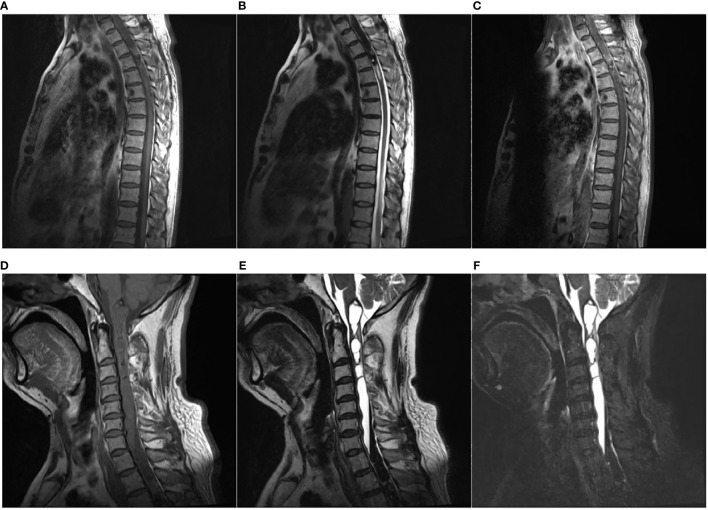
Magnetic resonance imaging (MRI). (A–C) An intramedullary tumor was located at C6–T4 with mixture of iso- and hypointensity on sagittal T1-weighted image (T1WI) (A), non-homogeneous hypointensity on sagittal T2-weighted image (T2WI) (B), and slightly non-homogeneous enhancement on sagittal T1WI with gadolinium (C). (D, E) Long T1 [(D), sagittal] and long T2 [(E), sagittal] signals with increased signal intensity of fat-suppressed images [(F), sagittal] at the level between medulla and C6 suggestive of syringomyelia at the proximal end of the lesion.
